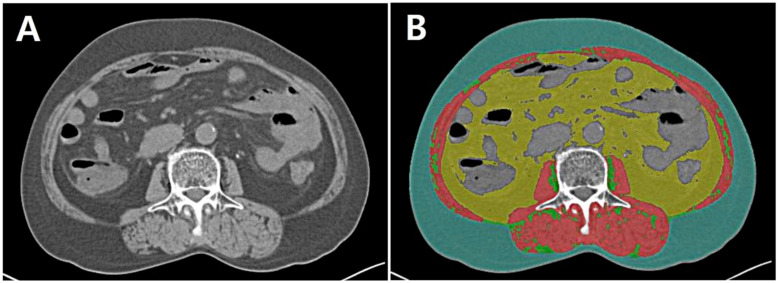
Cross-sectional abdominal computed tomography (CT) images at the L3 level. (A) Unenhanced (B) CT image with four body composition parameters applied using AutoMATiCA software (skeletal muscle area, red; visceral adipose tissue area, yellow; subcutaneous adipose tissue area, blue; intermuscular adipose tissue, green).
